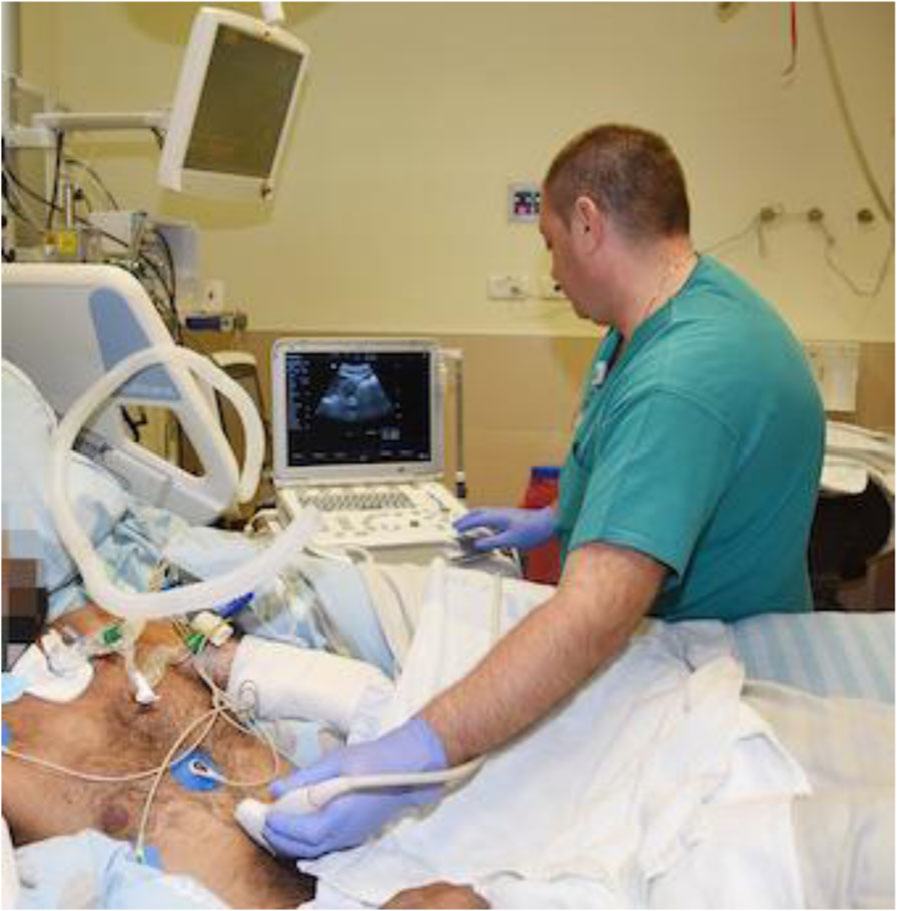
Ultrasound-guided residual stomach volume and nasogastric (NG) tube placement assessments performed by ICU nurse staff
